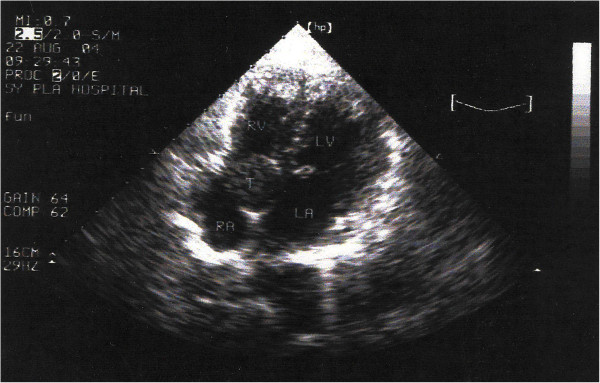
Transthoracic echocardiography showing a 3.2 × 2.7 cm mass in the RA attached to interatrial septum. (*LA* = left atrium; *LV* = left ventricle; *RA* = right atrium; *RV* = right ventricle; *T* = tumor).
